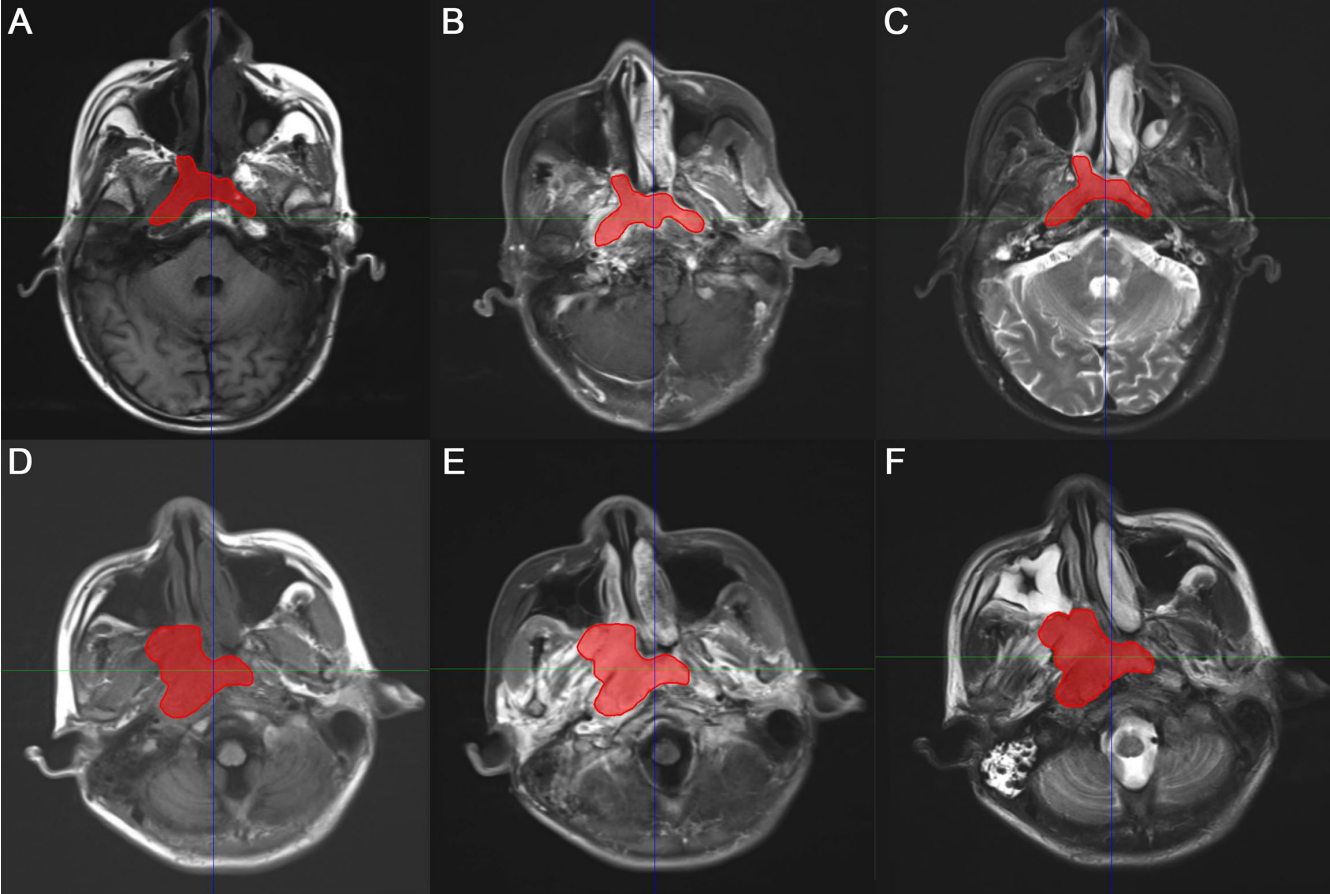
Representative two examples of contoured gross tumor volume (GTV) on multiple-sequence MRI. (A, D) T1-weighted, (B, E) T1 contrast, (C, F) T2-weighted MRI for patient 1 and 2, respectively.
